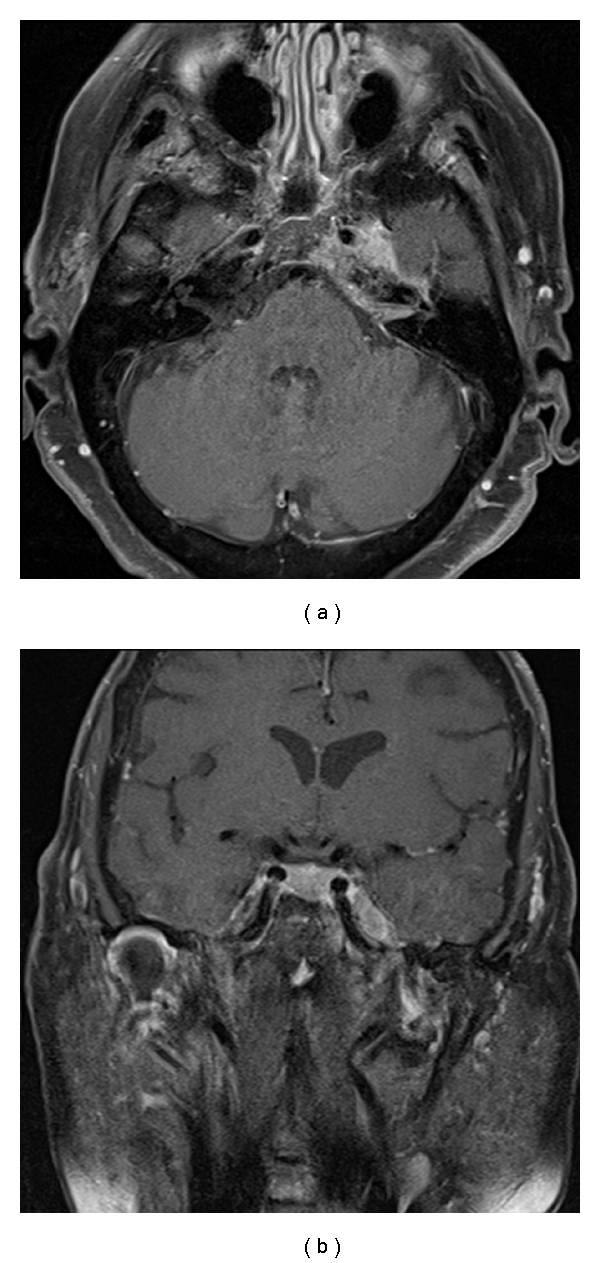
Axial and coronal T1-weighted MR imaging of the brain with gadolinium enhancement demonstrating a heterogeneously enhancing left petrous apex mass with extension into the adjacent middle cranial fossa and cerebellar pontine angle.
